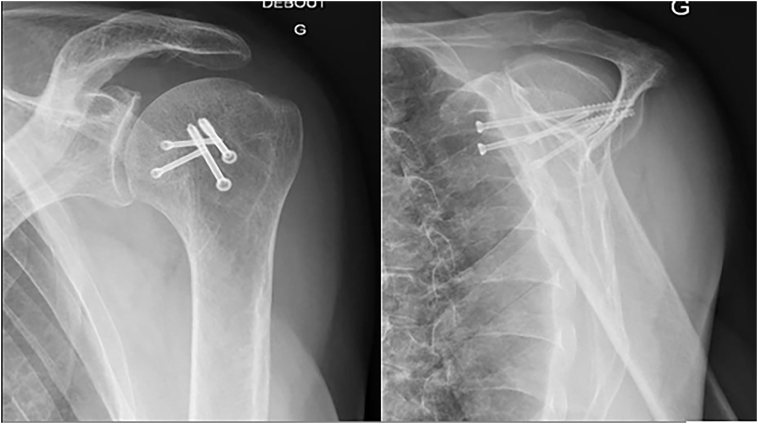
14 months follow-up X-ray left shoulder.
